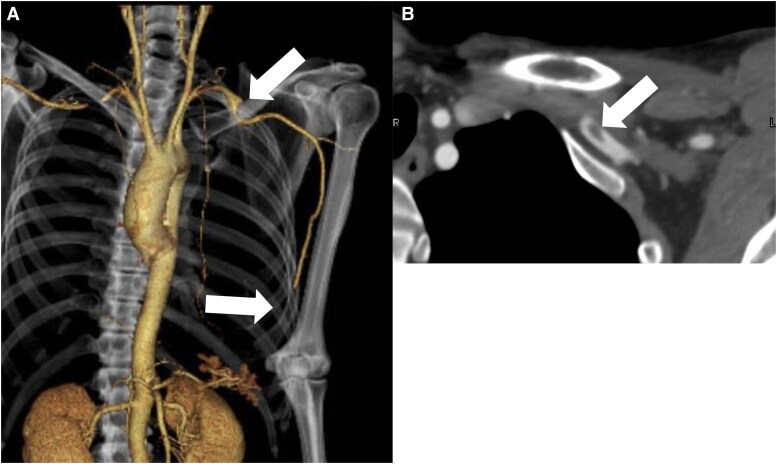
Contrast-enhanced computed tomography angiography revealed an aneurysmal change of the left subclavian artery with thrombus and a distal occlusion beyond the brachial artery.
